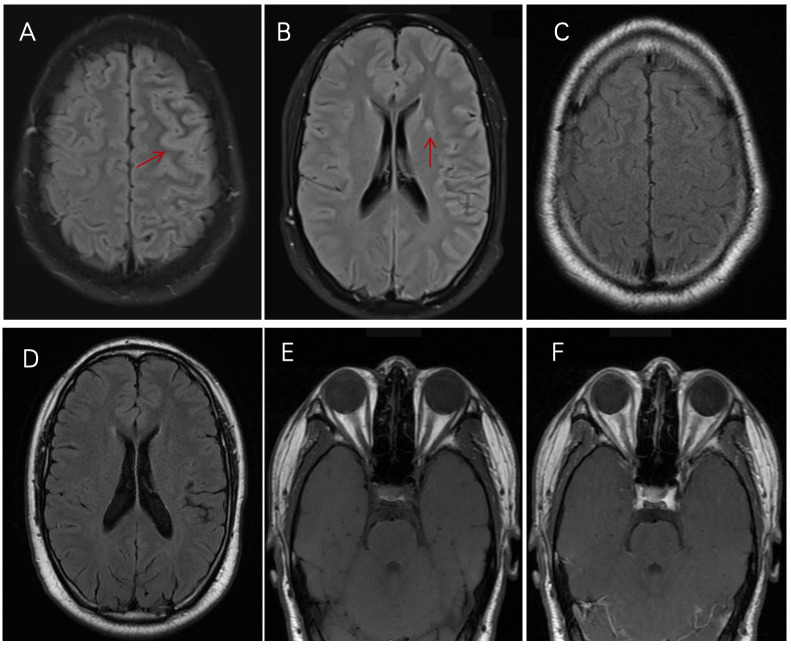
Magnetic resonance imaging. (A) Swelling of the left cerebral gyrus on FLAIR. (B) Hypersignal adjacent to the left lateral ventricle on FLAIR. (C, D) After antiviral treatment, the swelling of the gyri and the hyperintensity in the white matter on FLAIR sequences have both returned to normal. (E, F) No abnormalities of the optic nerve were observed on both T1 and T1-enhanced sequences.
